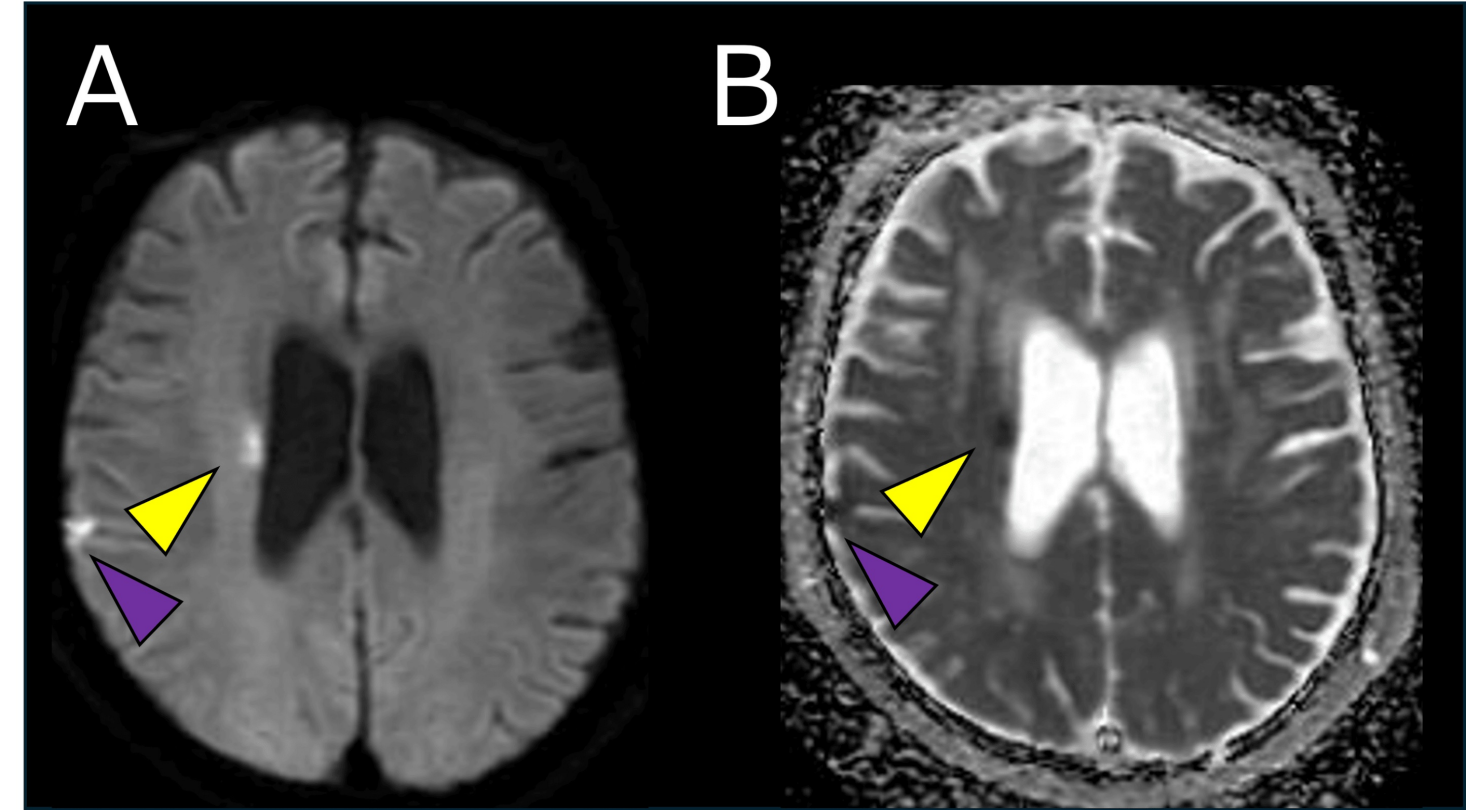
Brain MRI at Admission. Diffusion-weighted imaging (A) revealed high signal intensity in the right lateral striate artery territory, with corresponding low values on apparent diffusion coefficient mapping (B) (yellow arrowheads). The high signal observed in the right cerebral cortex on diffusion-weighted imaging (A) and the corresponding low value on the apparent diffusion coefficient map (B) appeared hypointense on the T2-weighted image (not shown), suggesting hemorrhage (purple arrowheads). MRI: magnetic resonance imaging.
